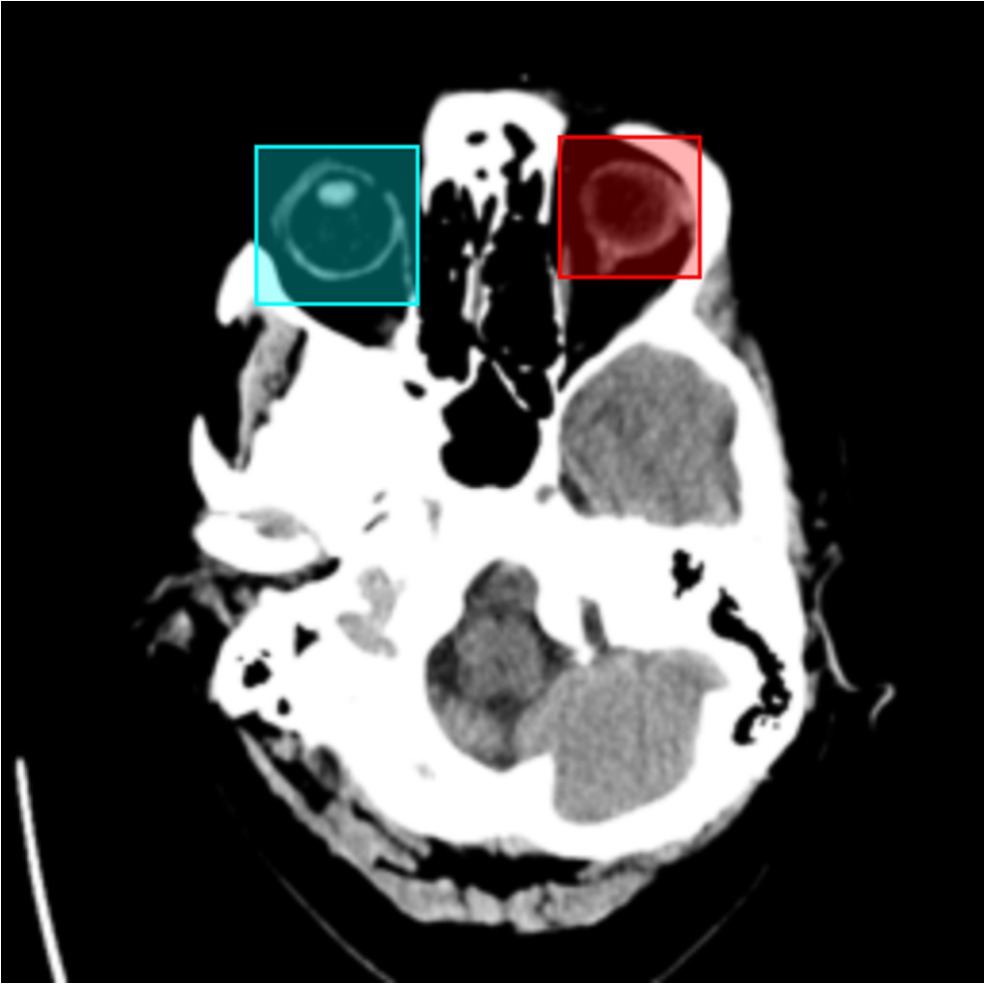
Simultaneous object detection of lens and globe in a representative axial head computed tomography (CT) examination slice
